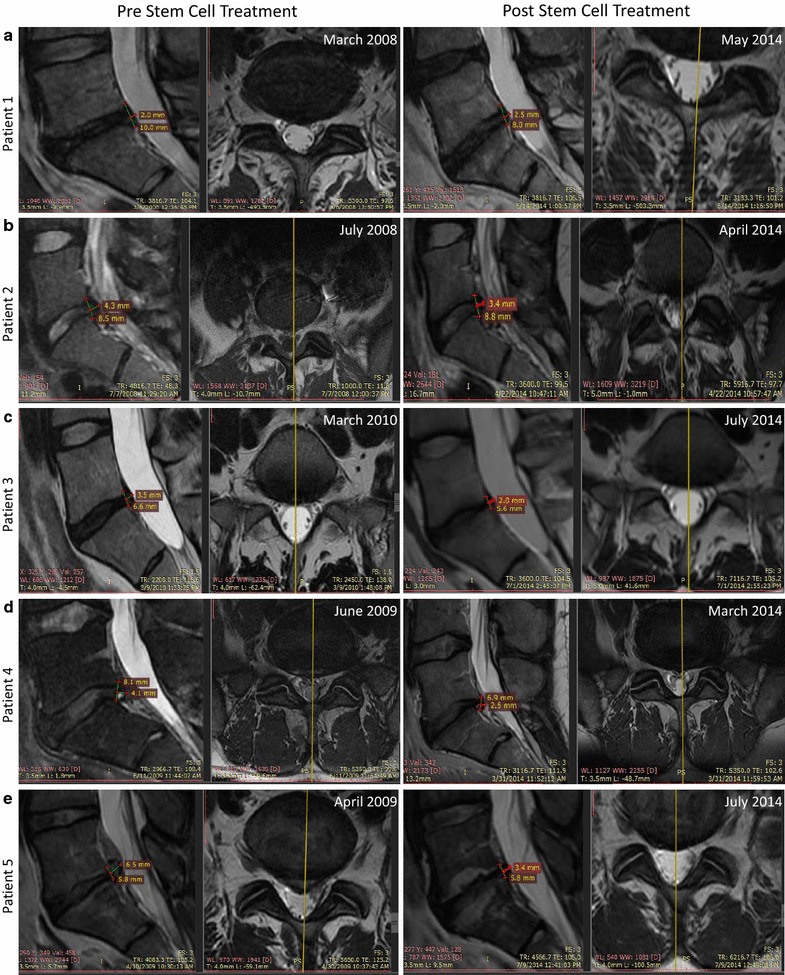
Patients MRI pre- and post- autologous, hypoxic cultured MSCs intra-discal injection. MRI pre- (*left panels*) and post- (*right panels*) stem cell treatment are shown for patient 1 (a), patient 2 (b), patient 3 (c), patient 4 (d), and patient 5 (e). Measurements of the posterior disc height and protrusion size at the L5–S1 level are indicated on each image as well as the date at which the MRI imaging was performed
